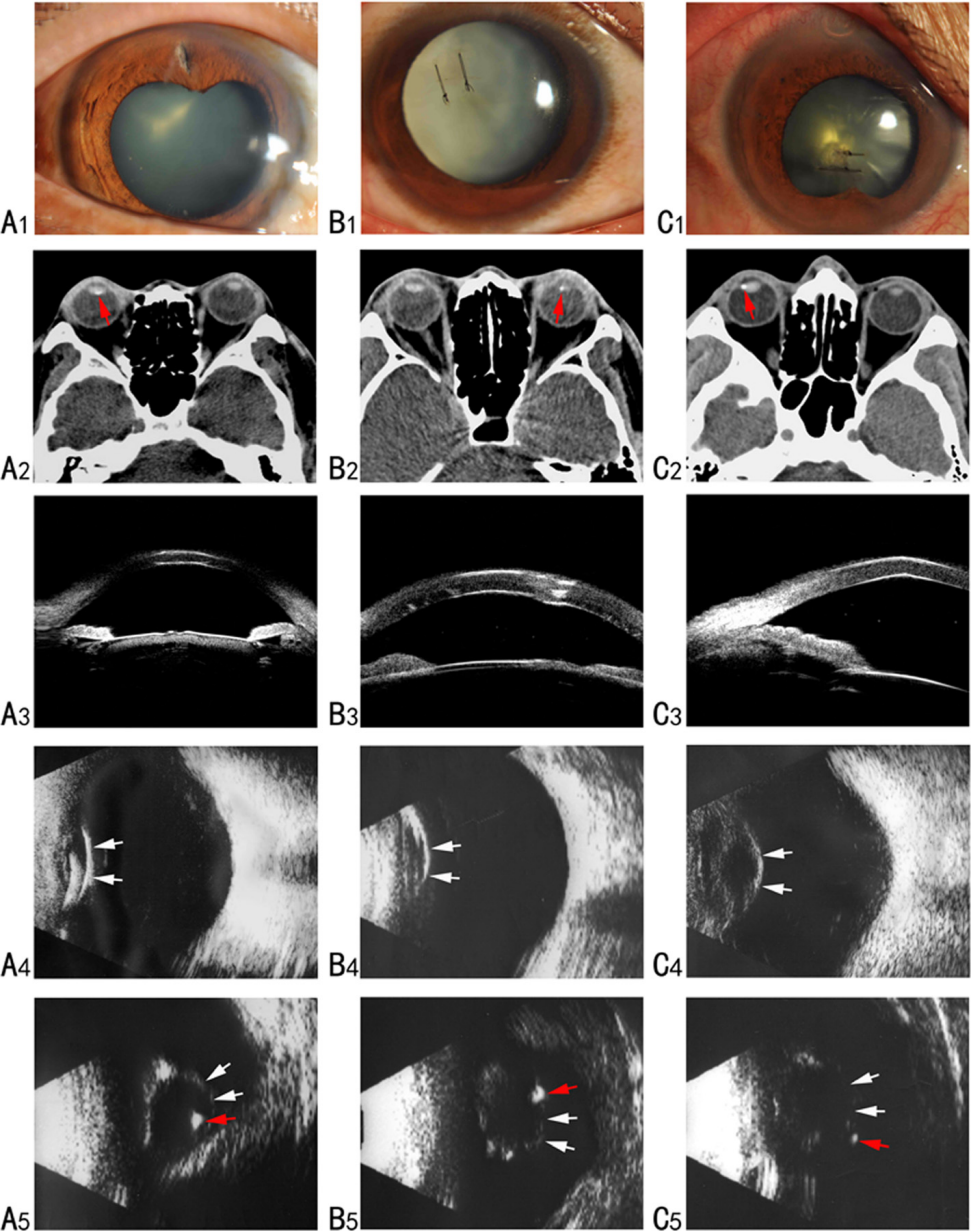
Preoperative photographs of the three patients. a Case 1, b case 2, c case 3. Slit-lamp photographs showing corneal laceration and lens opacity (a1-c1). CT scanning revealed intralenticular foreign bodies red arrow, a2-c2). UBM exam failed to detect any IOFB in the anterior segment (a3-c3). Axial scanning of B-scan ultrasonography shows the echo of the posterior surface of the lens (white arrow), with an abnormal echo in the posterior lens in case 1 (a4) but no evident IOFB in case 2 (b4) and case 3 (c4). Transverse scanning of B-scan ultrasonography clearly showed the position between the IOFB (red arrow) and the posterior lens capsule (white arrow)
